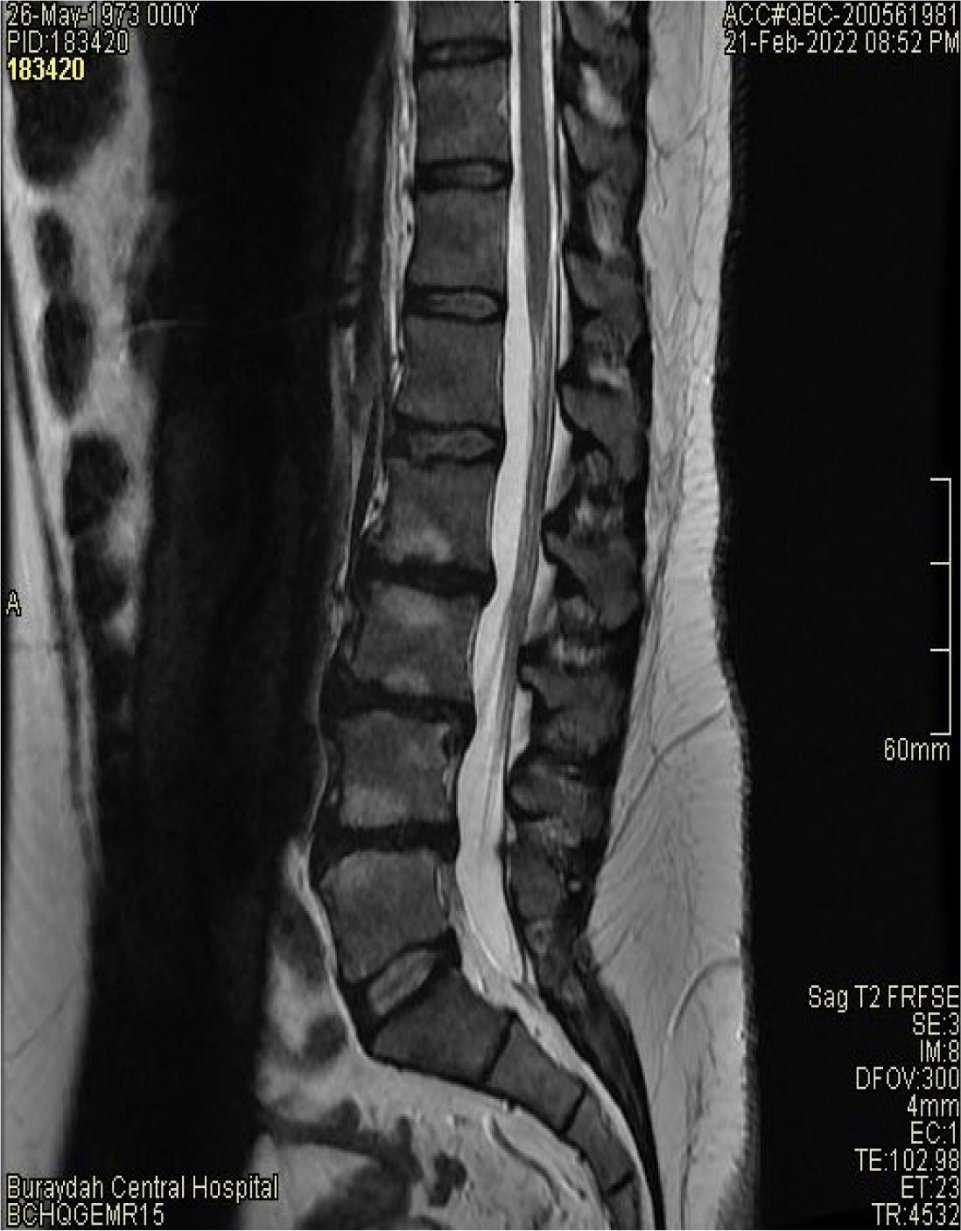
MRI of the lumbar spine after treatment showing reduced bulging of discs between L5 and L2
